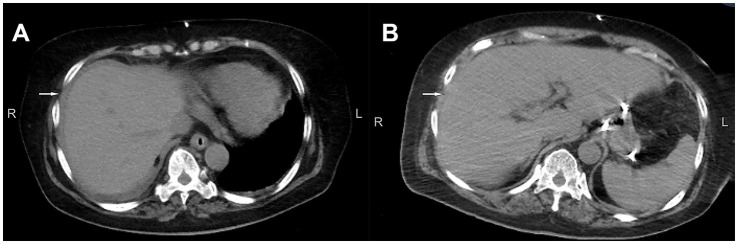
Abdominal CT scan. (A) CT on admission indicated a small amount of fluid around the liver. (B) On the seventh day of admission, CT showed that the fluid accumulation around the liver had disappeared. CT, computed tomography.
